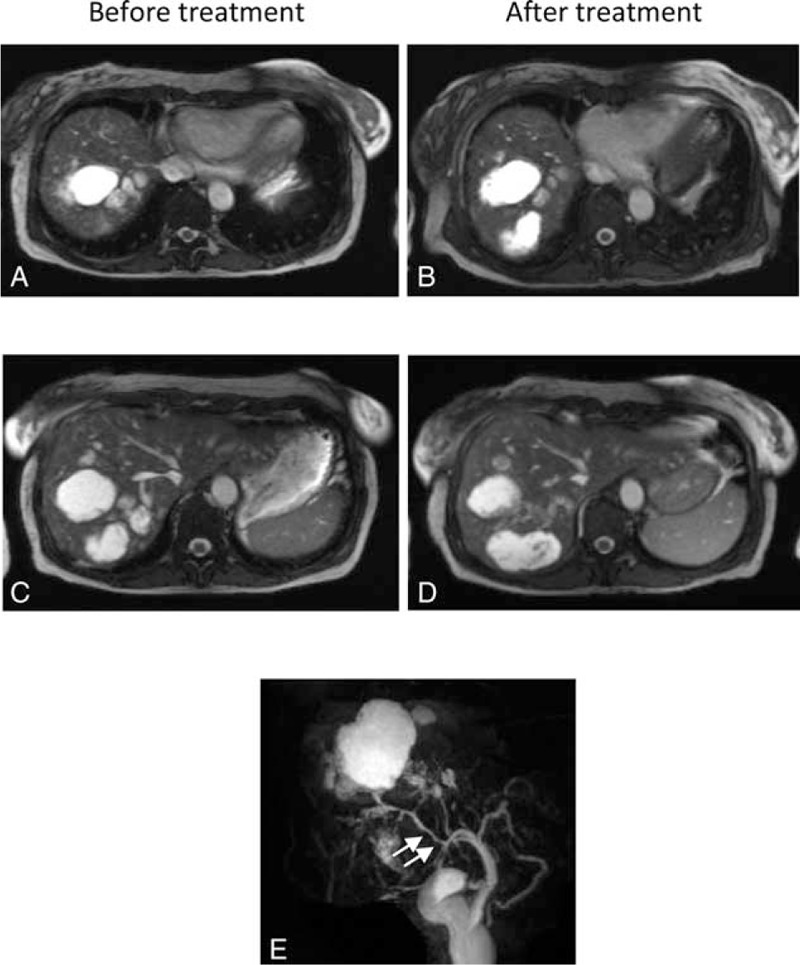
Liver MRI images before and after bevacizumab treatment. (A, B) axial balanced fast-field echo-weighted MRI images obtained before bevacizumab treatment demonstrate multiple bilomas. (C) MR cholangiography images show multiple areas with irregularities of the bile ducts arrows. (D, E) after 6 courses of bevacizumab, liver MRI images revealed an increase in the size and number of bilomas and persistence of the biliary irregularities. MRI = magnetic resonance imaging.
